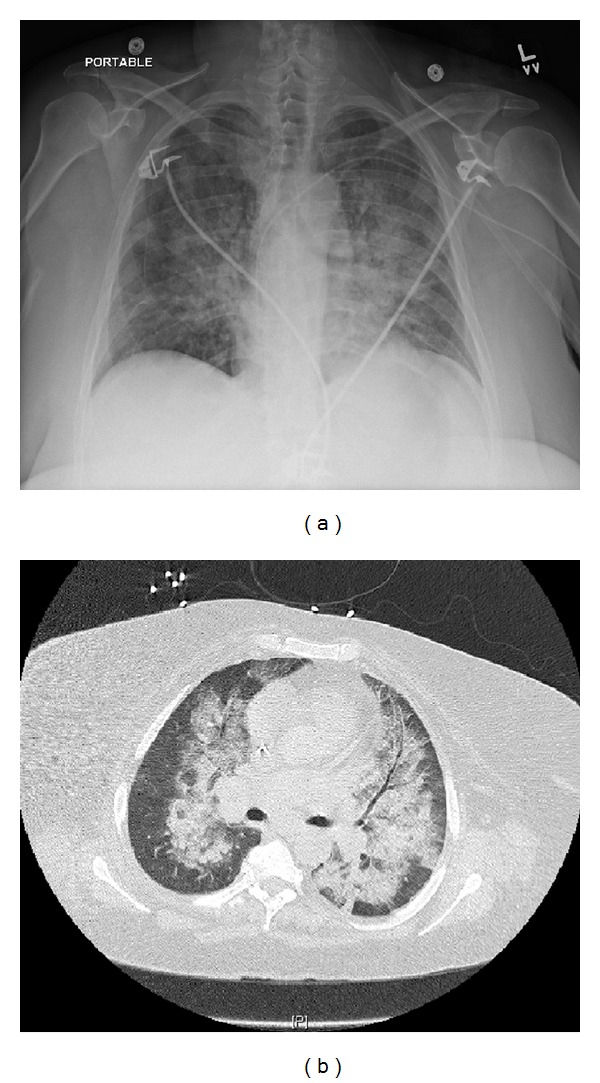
Chest X-ray (a) and computed tomography (b) demonstrating bilateral pulmonary infiltrates.
